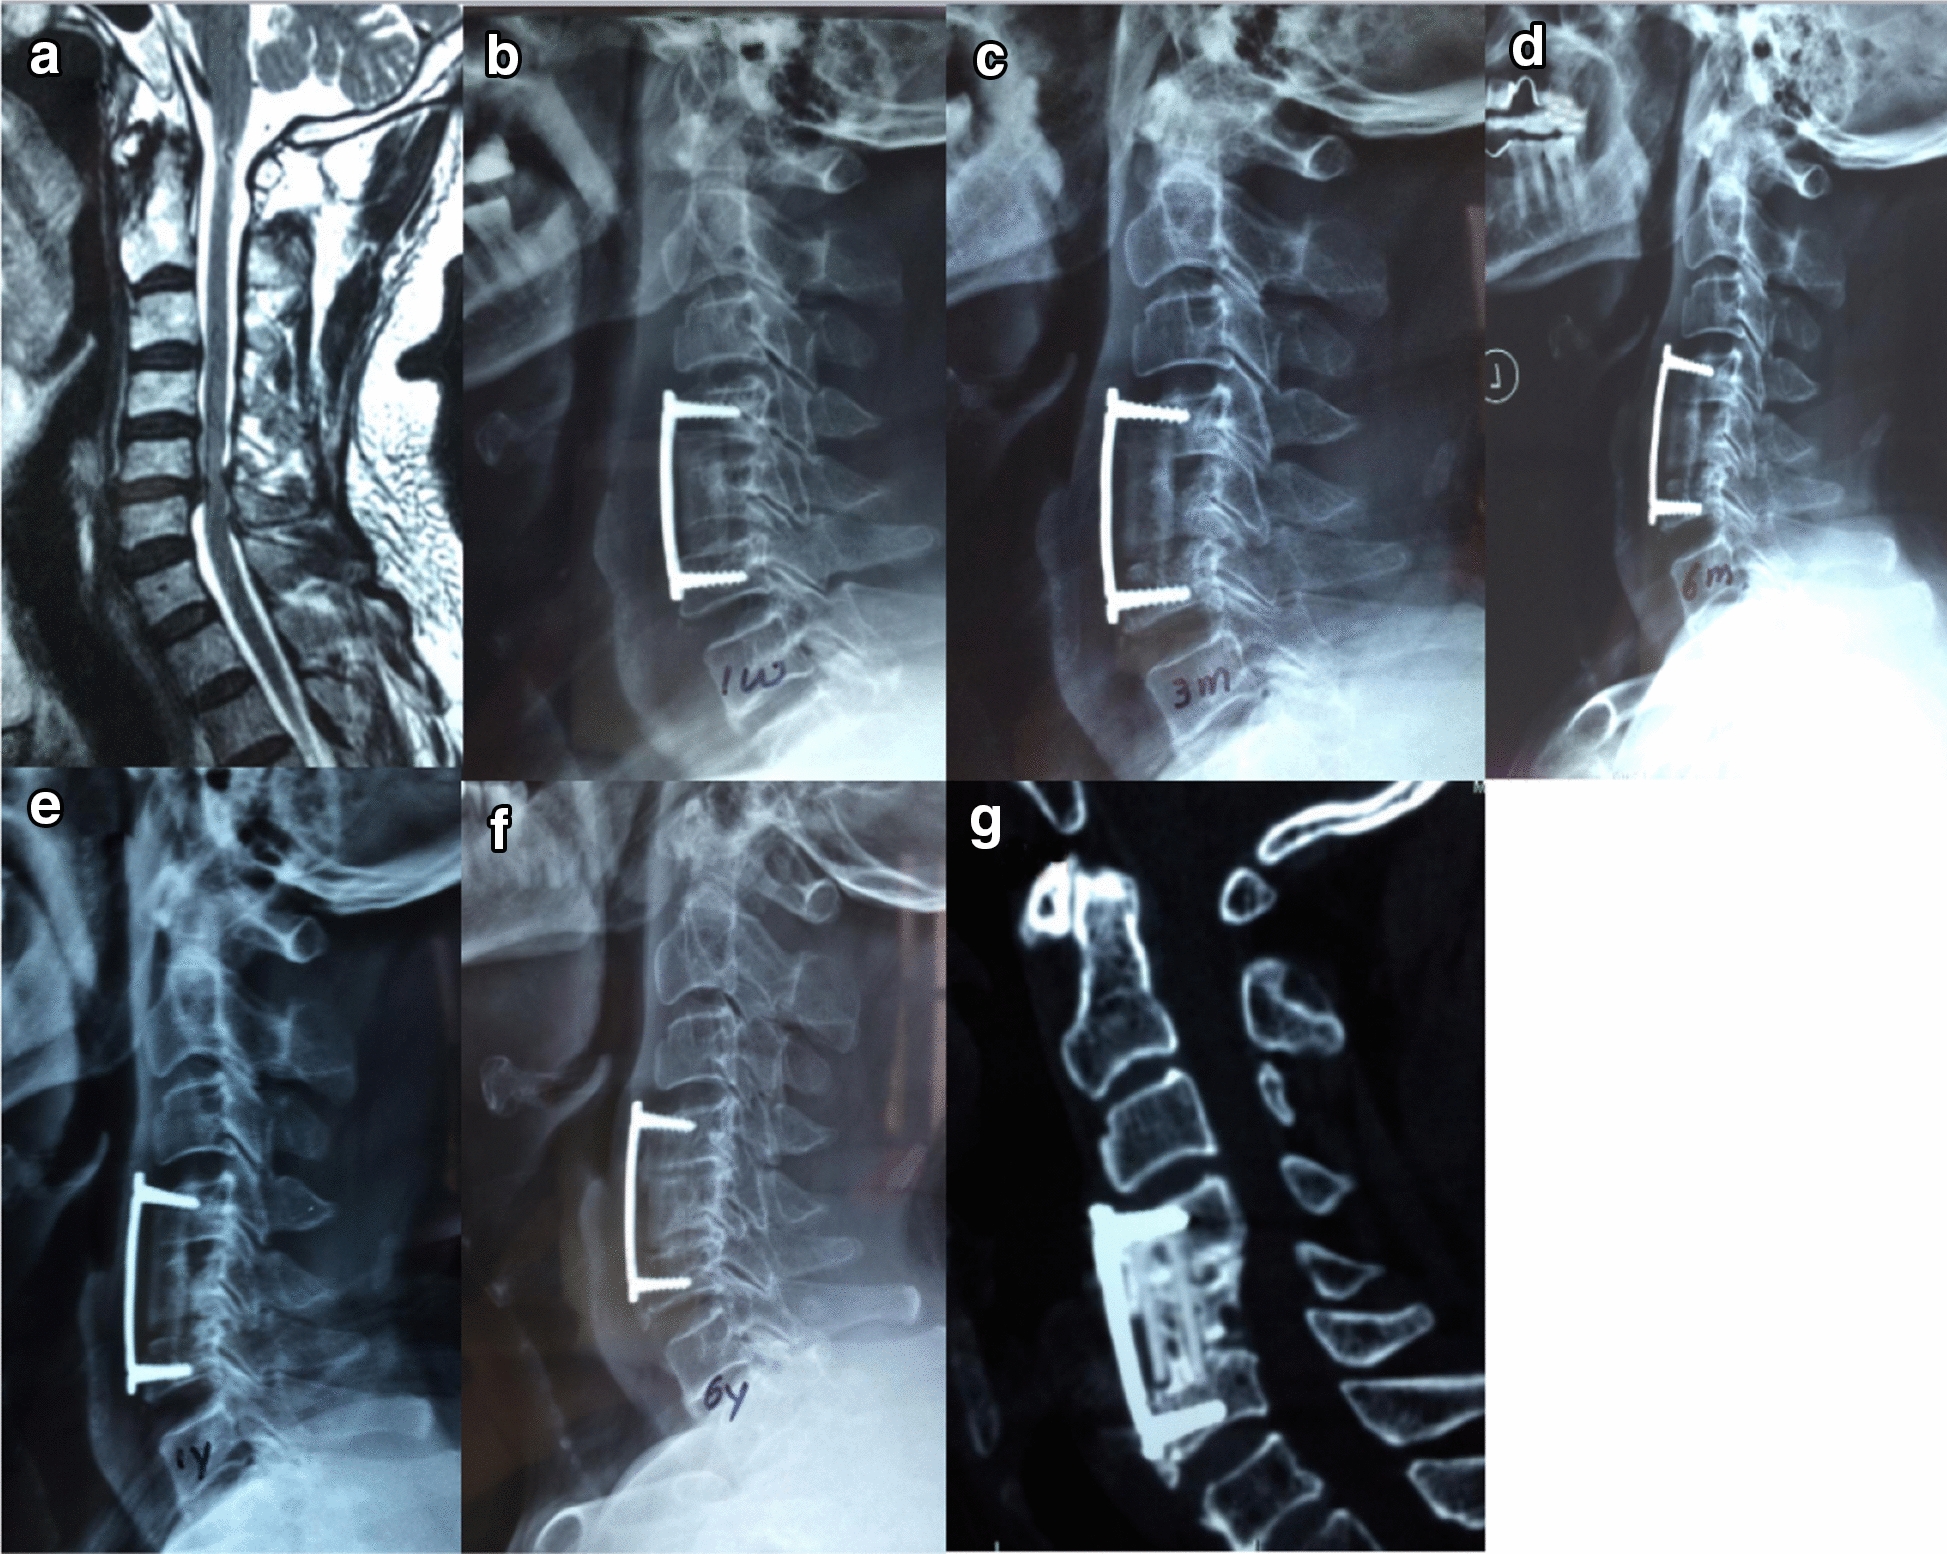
Type c bone fusion. a–f A 73-year-old man whose preoperative cervical MRI (a) revealed C5/6 disc herniation underwent 1-level corpectomy with an n-HA/PA66 strut (b). Lateral X-ray films indicated bony fusion of the autogenous bone granules filling the strut. Obvious subsidence and screw displacement were observed at the 3-month, 6-month, 12-month, and 6-year follow-up visits (c–f). 3-D CT at the 6-year follow-up (g) showed solid bone fusion, and the patient complained of no symptoms or signs
